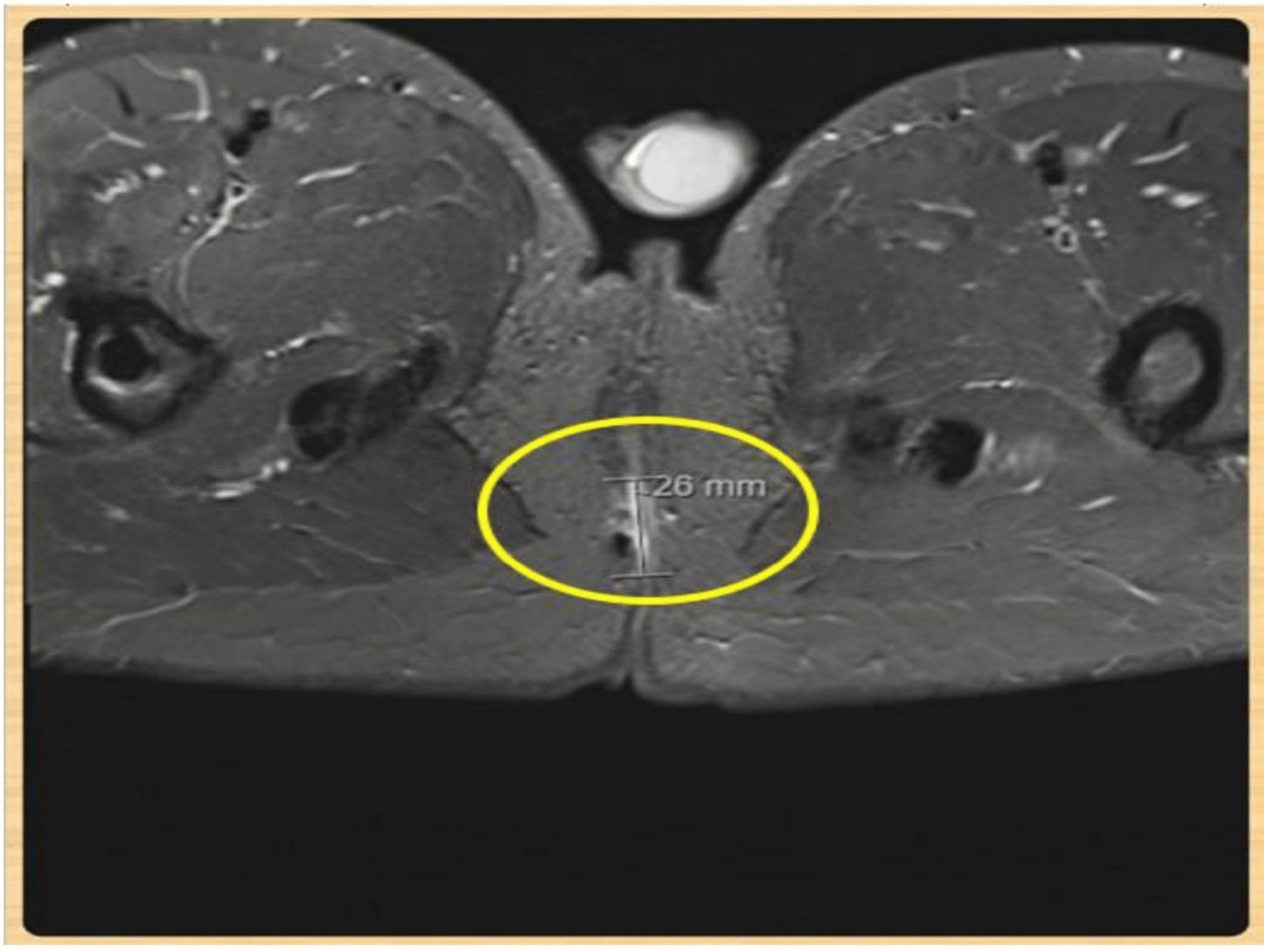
Pelvic MRI showing a 26-mm perineal injury accompanied by associated soft tissue disruption
